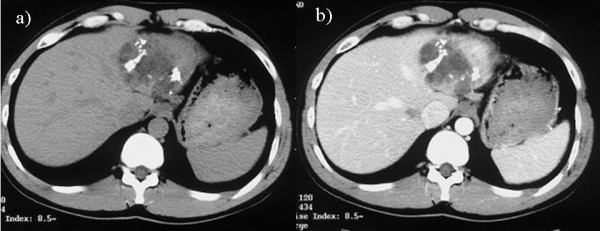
Abdominal CT findings in 2004. a) showing the unilocular cyst over 10 cm in diameter, increasing eruplioid calcification, septa and thickness of the wall within the cyst. b) the contrast was seen a little at the left side of the cyst.
